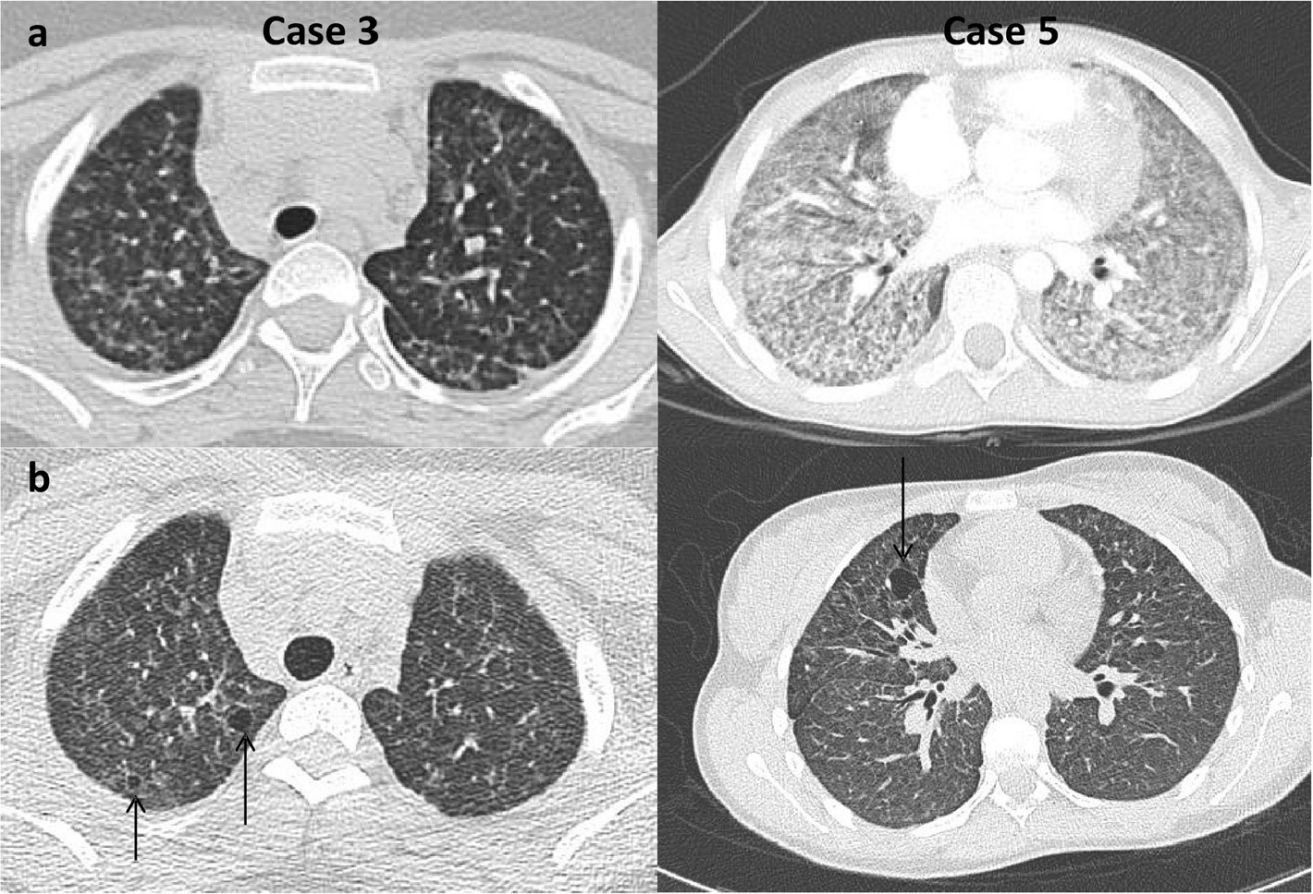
HRCT scan in “persistent diffuse interstitial subset” ICEP patients. (a) At diagnosis: in cases 3 and 5, diffuse ground glass opacities, reticulonodular syndrome. (b) Last HRCT scan showing diffuse ground-glass opacities with development of thin-walled cysts in cases 3 and 5.
